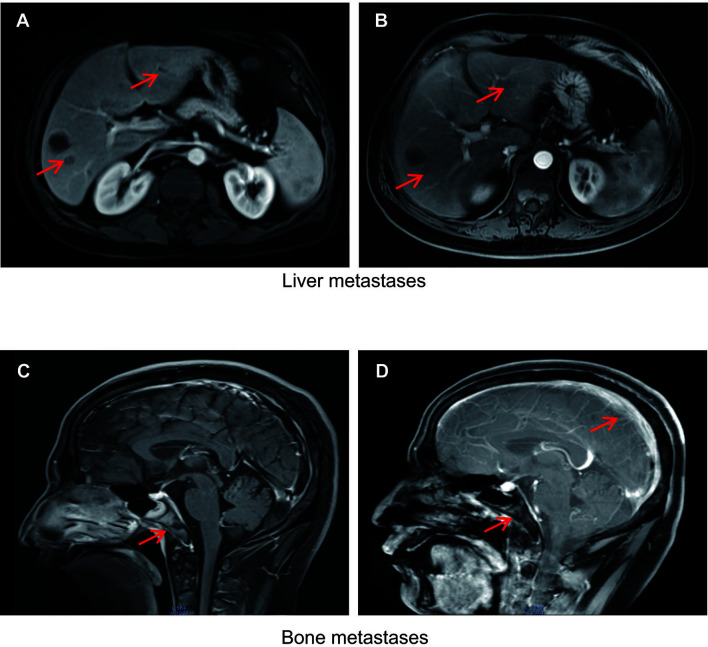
(A) Liver metastases detected using magnetic resonance imaging (MRI) in November 2019. (B) Response of liver metastases detected using MRI in January 2020 after treatment with etoposide combined with cisplatin and continued ADT plus pembrolizumab. Metastases are indicated by red arrows. (C) Clivus and adjacent right sphenoid bone metastases detected by MRI in April 2020. (D) Meningeal clivus and adjacent right sphenoid metastases in November 2020. Metastases are indicated by red arrows.
